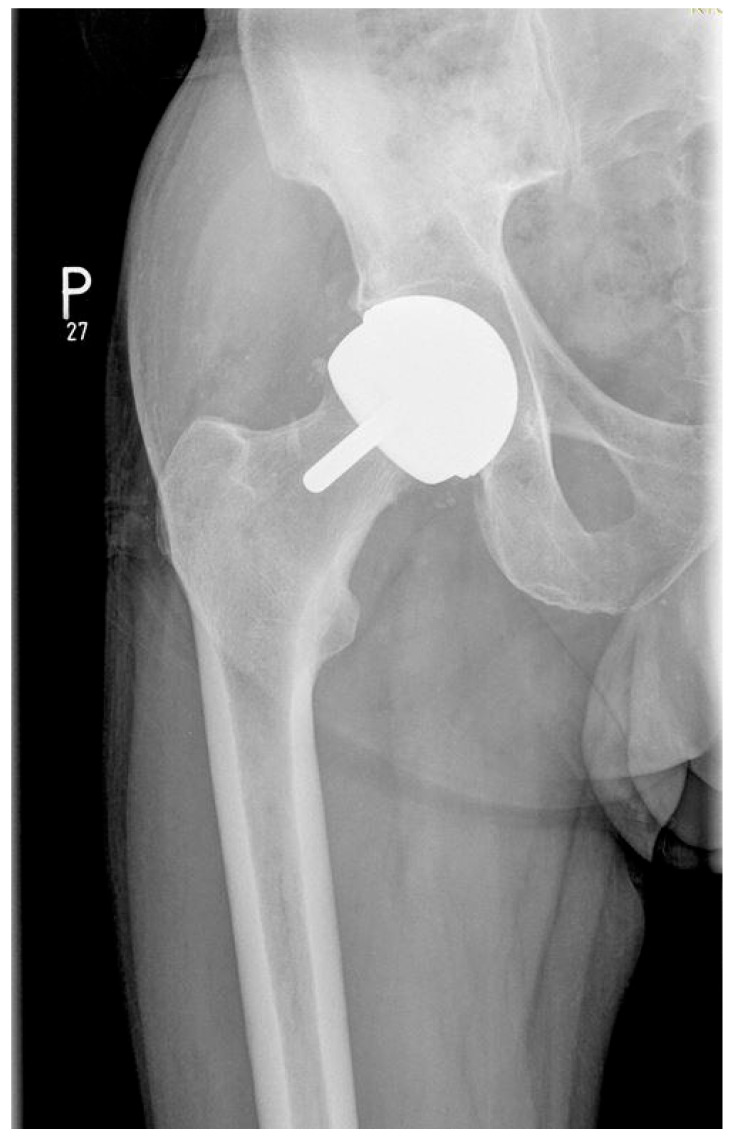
X-ray scan showing the hip operated with use of ReCap-Magnum (Biomet, Warsaw, IN, USA).
